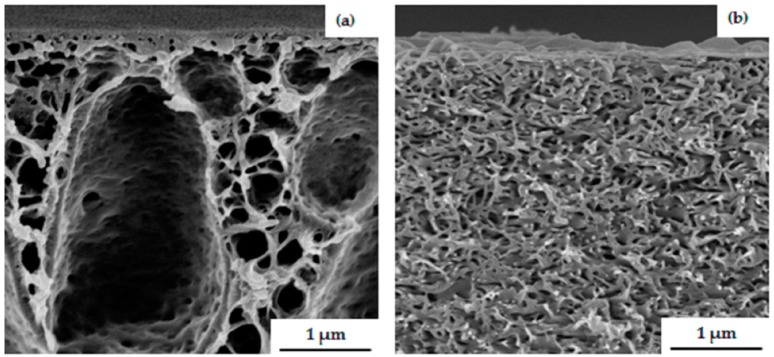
SEM images of the cross-section of the membrane prepared from 24 wt % PS_81_-PSMA_19_^170^ in THF/DMF 50/50 wt % at (a) 5 s, (b) 25 s time of evaporation.
